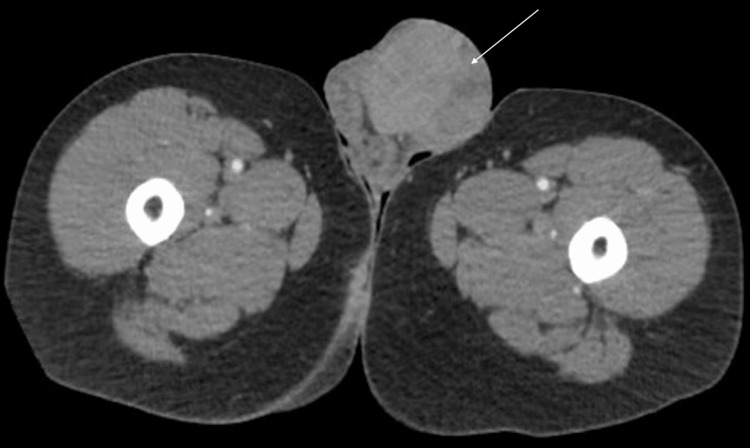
CT abdomen pelvis through the thigh demonstrating a large, left-sided scrotal mass (white arrow)
